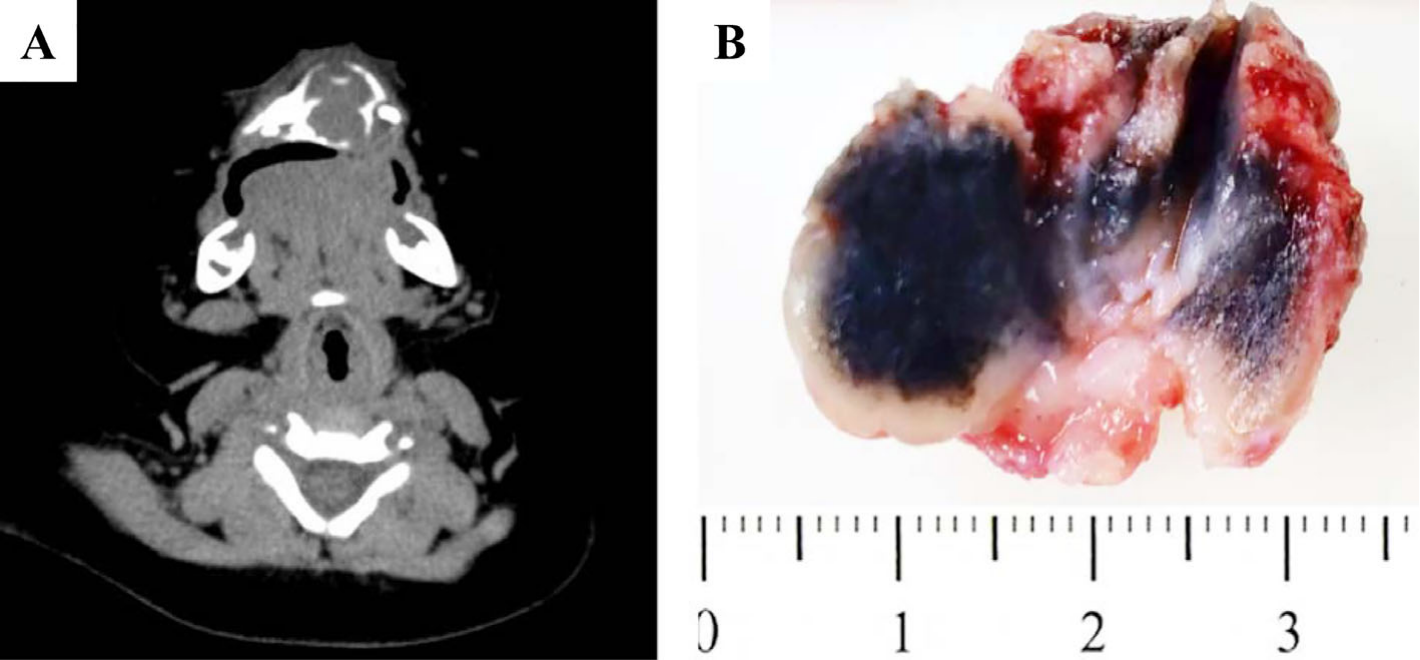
Gross pictures and radiological features of MNTI. (A) CT images demonstrated well-defined mass with lytic bone destruction and tooth germ affecting. (B) The tumor was firm, unencapsulated and the cut-surface was pigmented.
